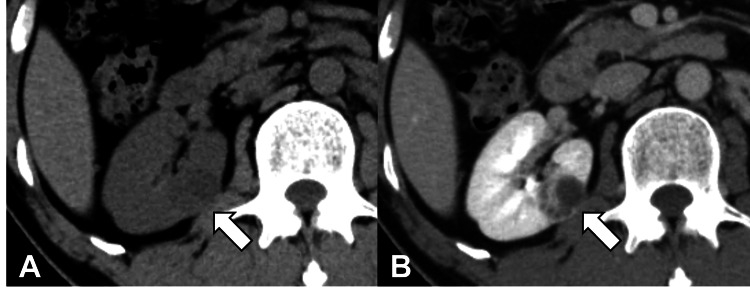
CT images of a 57-year-old woman with endophytic clear cell RCC A: unenhanced image; B: enhanced image in nephrographic phase A heterogeneous renal mass without deformation of the cortical outline is seen in the inner segment of the right kidney on UE-CT (arrows). Pathologic examination confirmed that the mass was a 2.0-cm clear cell RCC of the ENT type ENT: endophytic; RCC: renal cell carcinoma; UE-CT: unenhanced computed tomography
